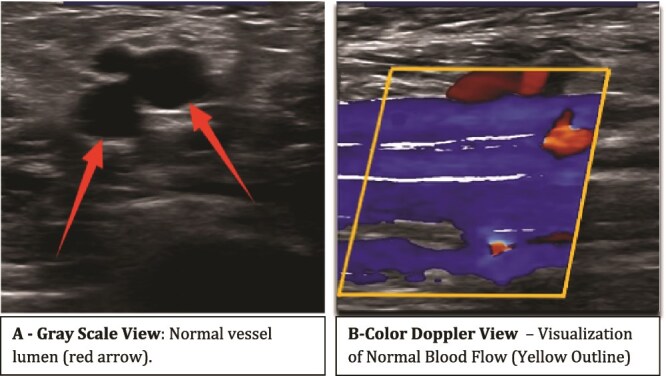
Doppler ultrasound of left leg vessels.
